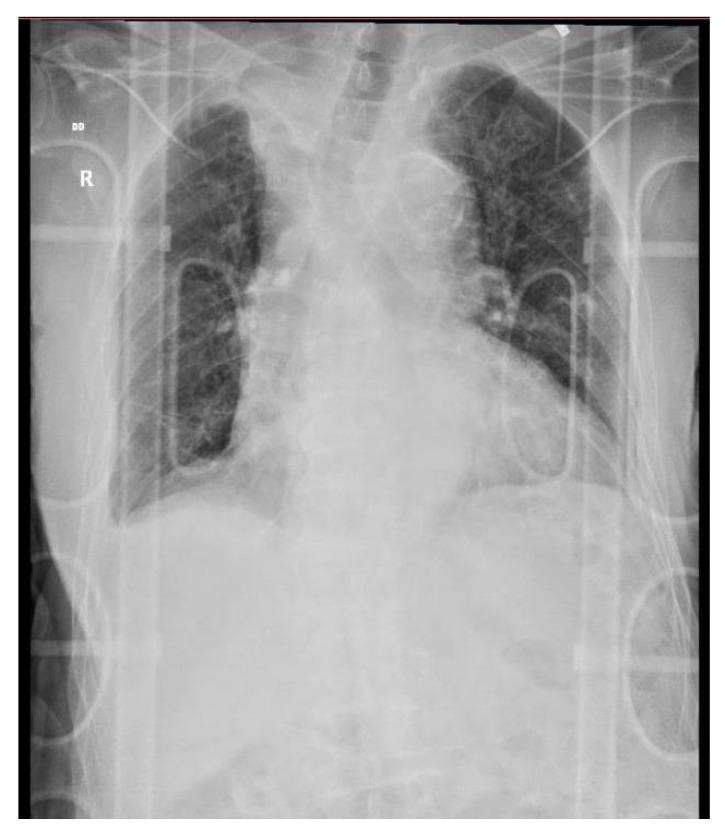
Chest X-ray upon arrival as per pre-surgery protocol, anteroposterior incidence. Description: diffuse, bilateral, reticular pattern. Possible right upper lobe atelectasis. Increased area of projection of the heart and mediastinum. Minimal right pleural effusion. No signs of rib fractures.
